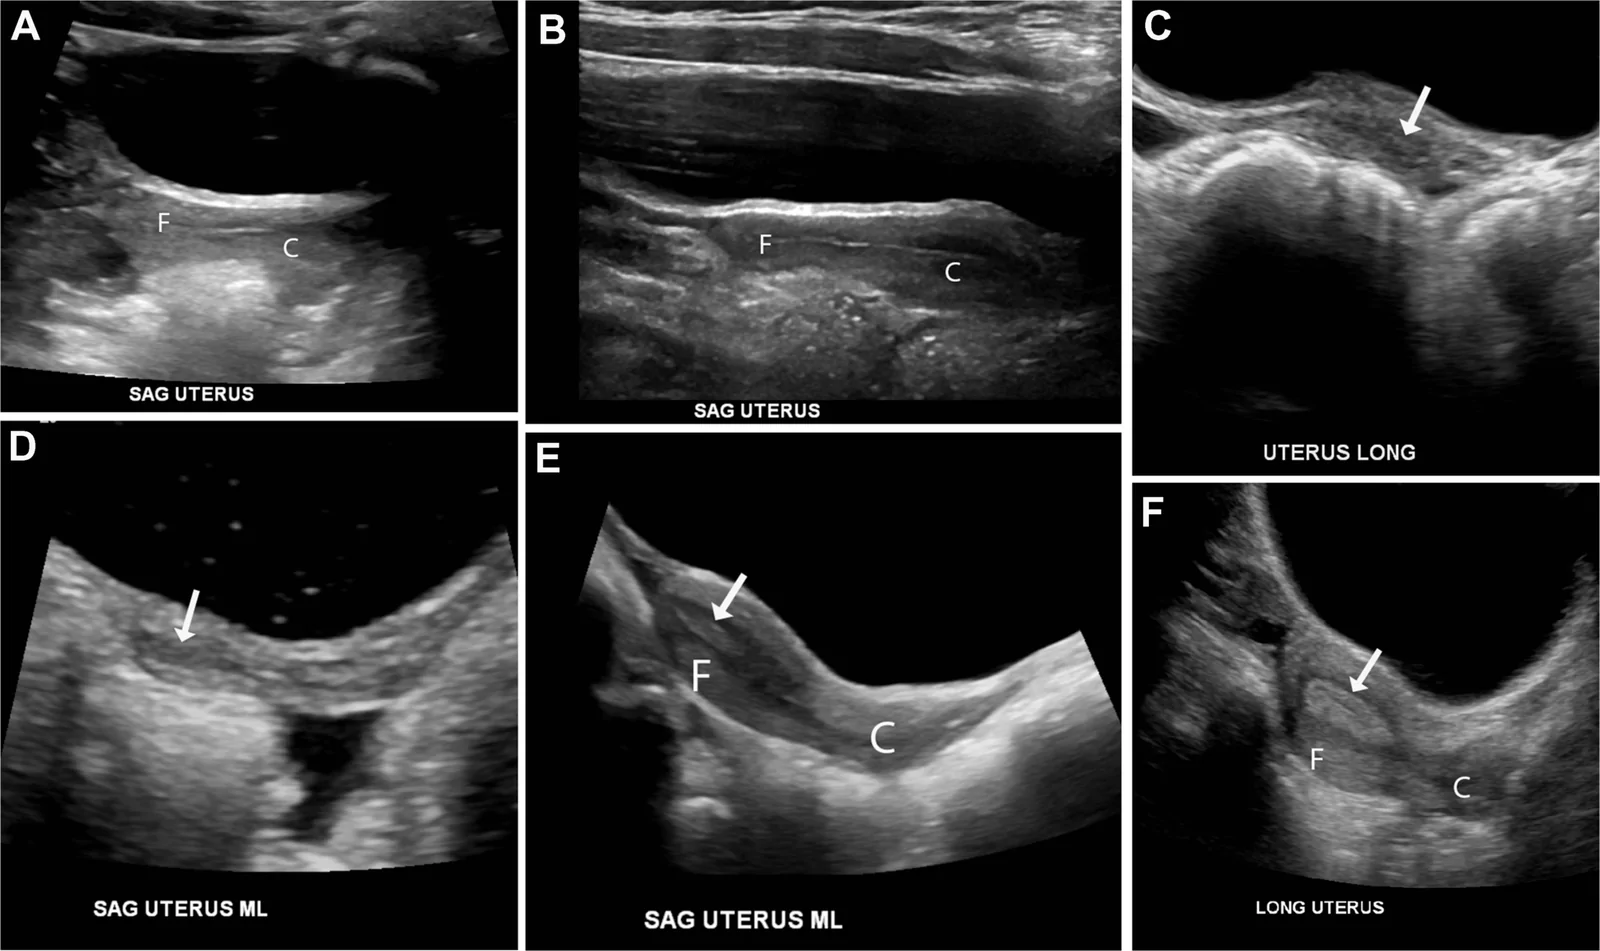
Normal appearances of the uterus over increasing ages. Longitudinal midline transabdominal pelvis ultrasound images show the sagittal appearance the uterus in several different patients at the following ages: (a) 6 months (3.2 cm long); (b) 9 months (3.2 cm long); (c) 4 years (1.8 cm long); (d) 9 years (3.2 cm long); (e) 12 years (6.8 cm long); and (f) 17 years (7.2 cm long). Note that in infancy (a, b), the uterus has a tubular morphology and the ratio of the fundal (*F*) length to cervical (*C*) length is close to 1:1. In the pre-pubertal school age (c, d), the uterus is small with only a faintly visible fundal endometrial stripe. At puberty (e, f), the fundus (*F*) enlarges disproportionate to the cervix (*C*), and the endometrial stripe (*arrow*) thickens
